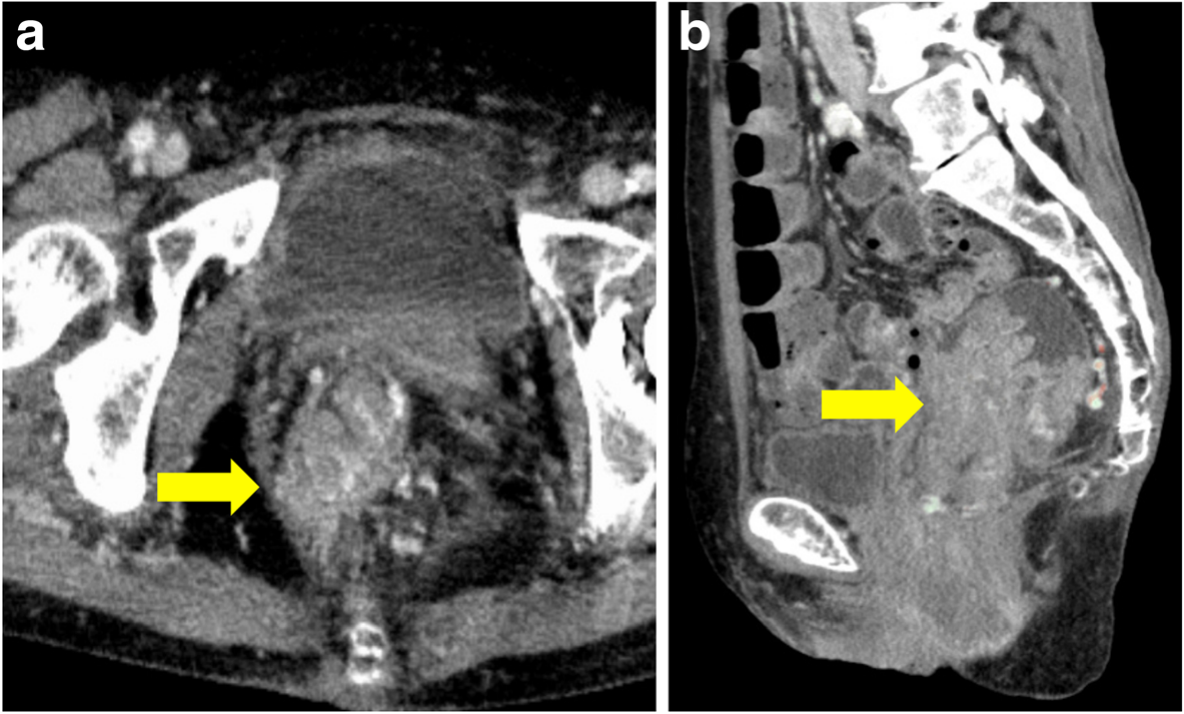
Computed tomography scan showing the lower rectum filled with a tumor measuring 7 × 6 cm, enhanced with contrast medium. The arrows pointing to the tumor. a axial plane, b sagittal plane
